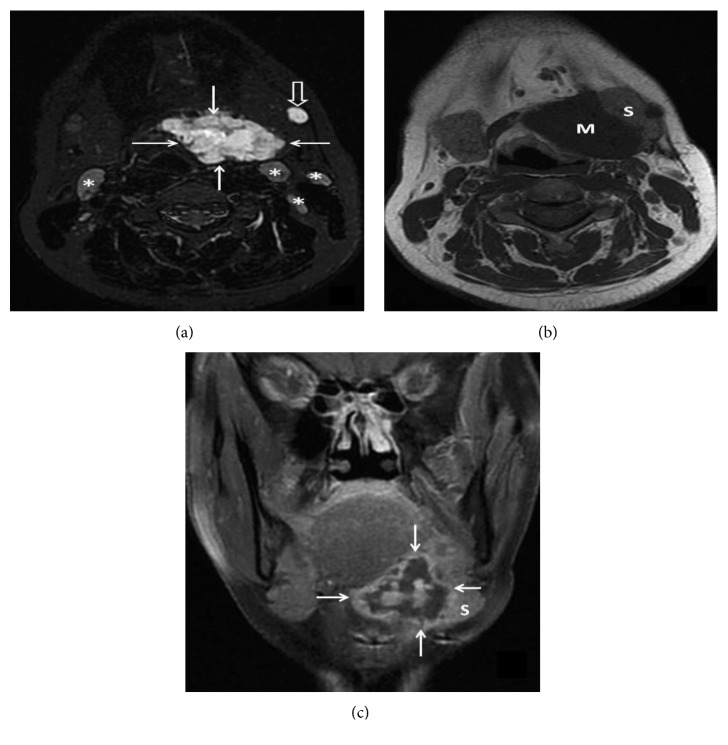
(a) Axial fat-sat T2 MRI image shows marked hyperintense mass with lobulated contours (arrows). There are metastatic lymph nodes within the left submandibular area (open arrow) and both sides of the neck lymph node chain (asterisk) with similar signal characteristics. (b) Axial T1 MRI image demonstrates effacement of the fat planes between the mass (M) and the left submandibular gland (S). (c) Coronal contrast-enhanced fat-sat T1 image reveals that the mass has an heterogeneous peripheral and central enhancement with some cystic-necrotic components (arrows).
